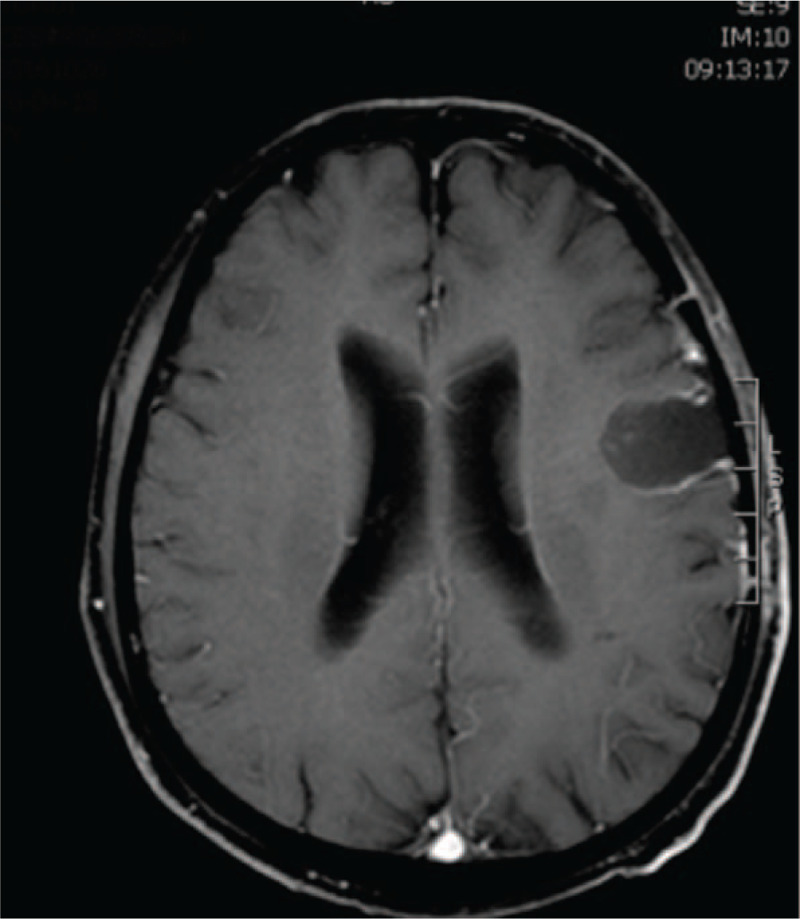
Follow-up MRI in Case 2. Six months after the operation, follow-up MRI showed no tumor recurrence.
